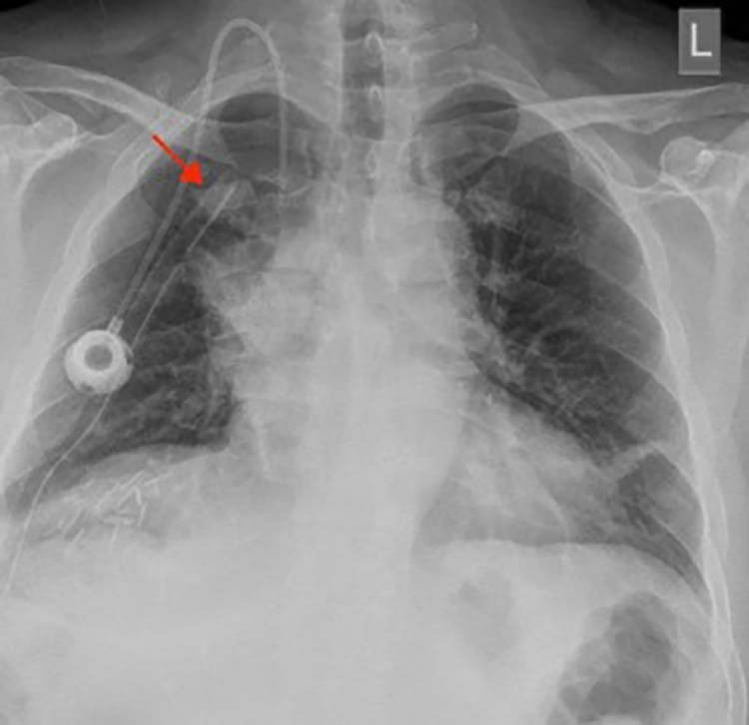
Chest X-ray (PA view) showing the right upper lobe mass. PA: posterior anterior.
